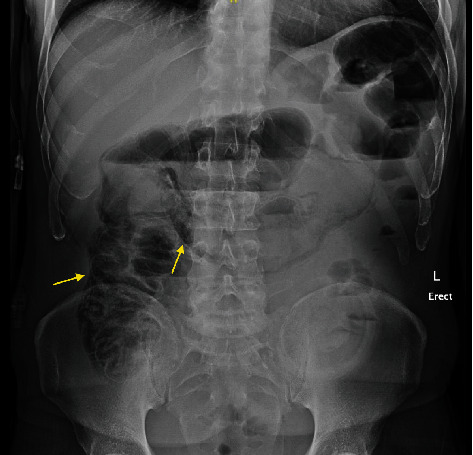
Abdominal X-ray demonstrating extensive pneumatosis intestinalis in the right and transverse colon and greater omentum (yellow arrows). End colostomy stoma bag is seen in the left lower quadrant.
